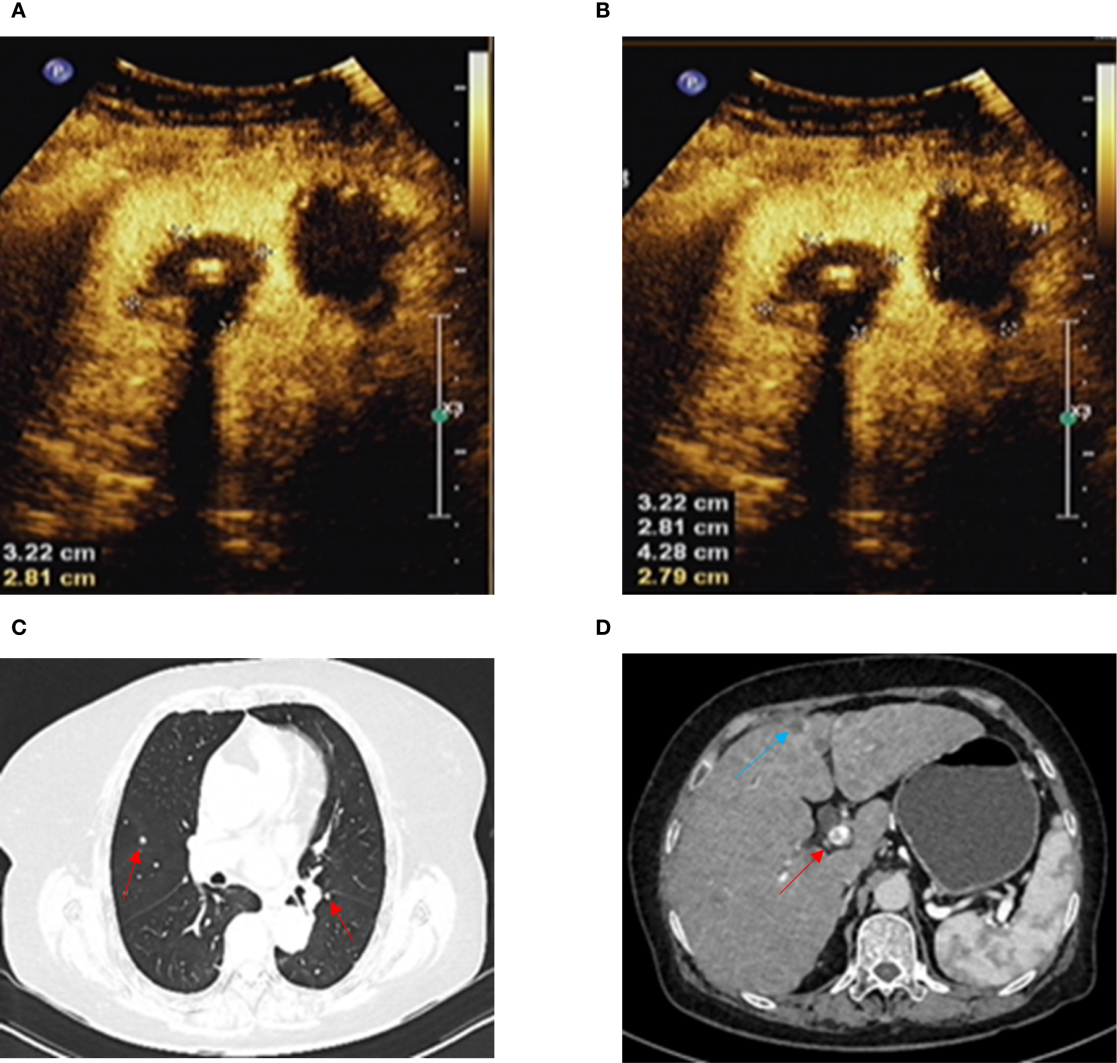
(A, B) Ultrasound contrast shows complete inactivation of liver metastases. (C) Chest-enhanced CT shows lung metastasis (red arrow). (D) Abdominal-enhanced CT shows liver metastasis (blue arrow) and portal vein thrombosis (red arrow).
